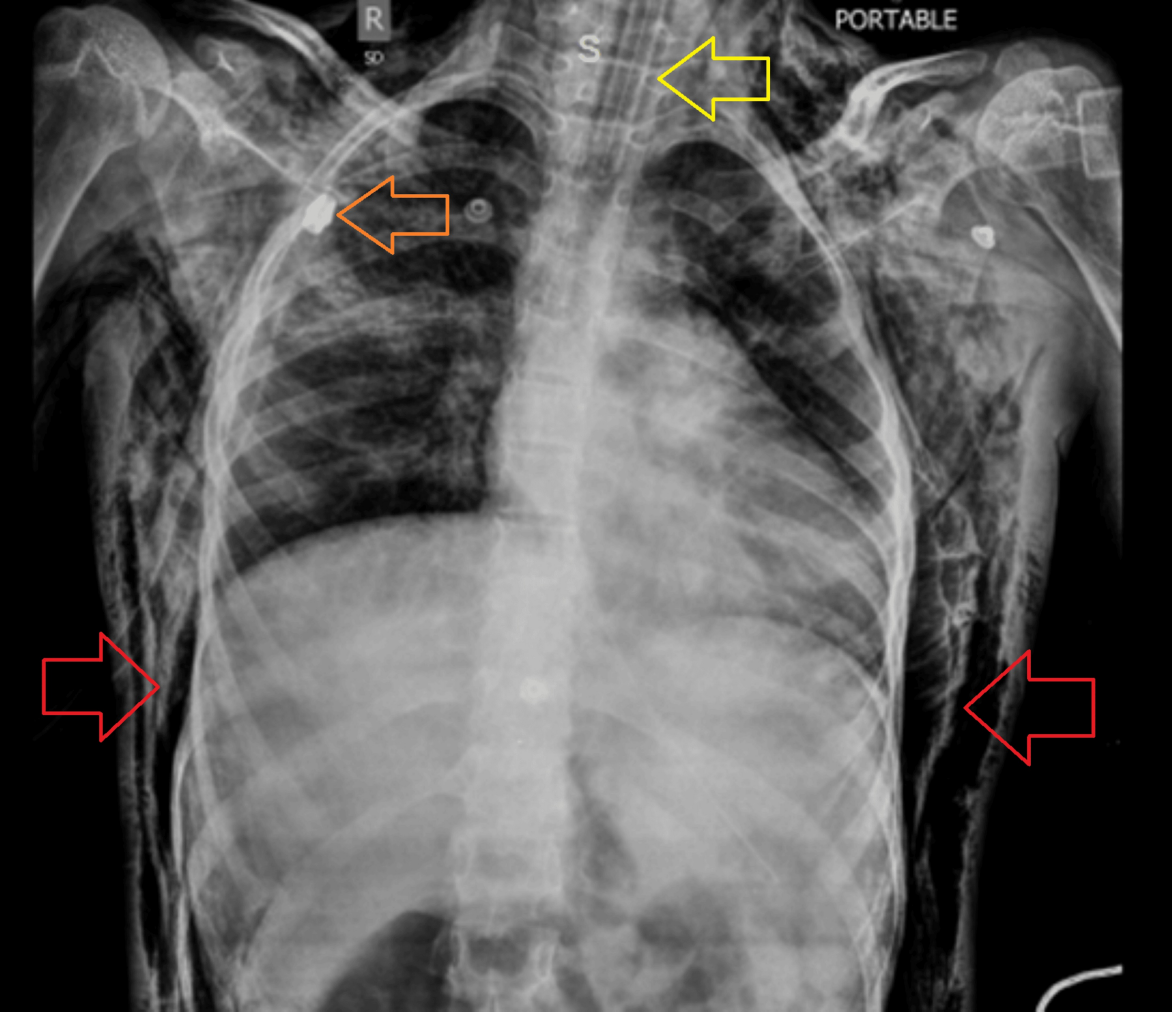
Chest X-ray immediately after passing the chest tube (orange arrow) along with bilateral subcutaneous emphysema along the chest wall (red arrow) with the endotracheal tube in place (yellow arrow)
